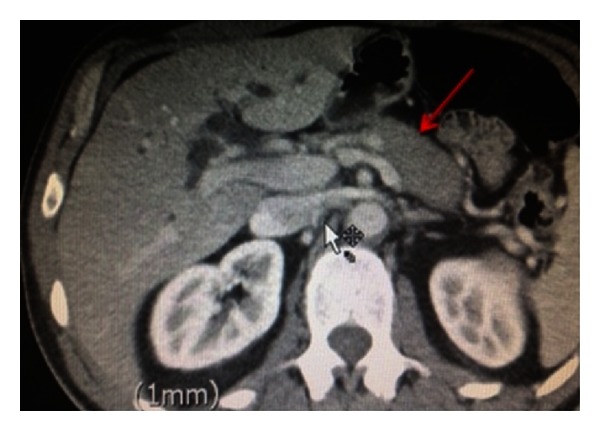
CT abdomen with “sausage-shaped” pancreas.
